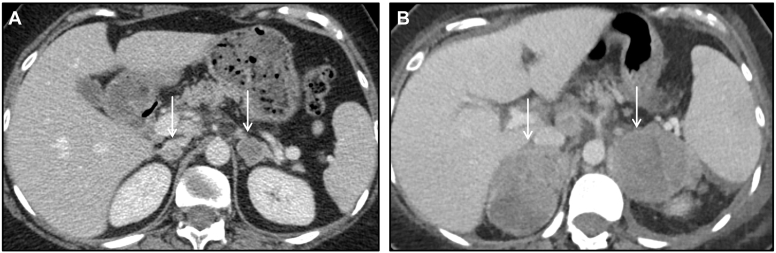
Bilateral adrenal metastases from melanoma. (*A*) Axial CT image with contrast showing bilateral adrenal lesions with heterogenous enhancement in a patient with known metastatic melanoma. (*B*) Axial CT with contrast 6 months later shows increase in size of the bilateral lesions, consistent with progressive metastatic disease. *CT* = computed tomography.
